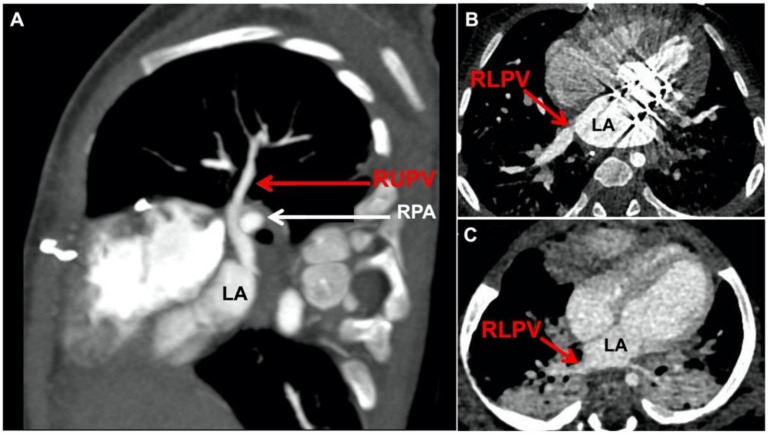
Right pulmonary veins (CT images). (A) Right upper pulmonary vein crosses over right pulmonary artery causing angulation as it passes across pericardial reflection into left atrium; (B) Right lower pulmonary vein stenosis involves more posteriorly directed pulmonary vein that angulates when crossing pericardial reflection; (C) Right lower pulmonary vein without stenosis has straight, less angulated course into left atrium. (RUPV = right upper pulmonary vein; RPA = right pulmonary artery; RLPV = right lower pulmonary vein; LA = left atrium).
